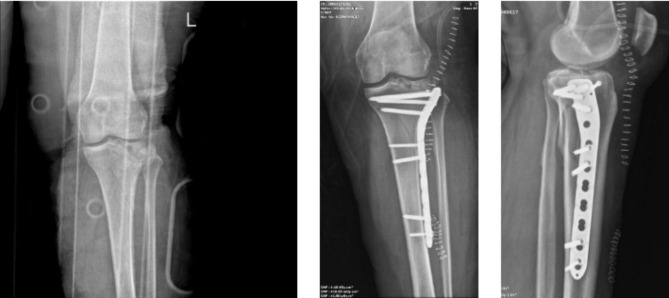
A 45-year-old male patient with tibial plateau fracture after falling from e-sccoter. (A) Preoperative antero-posterior radiography, (B) Postoperative antero-posterior radiography, (C) Postoperative lateral radiography
